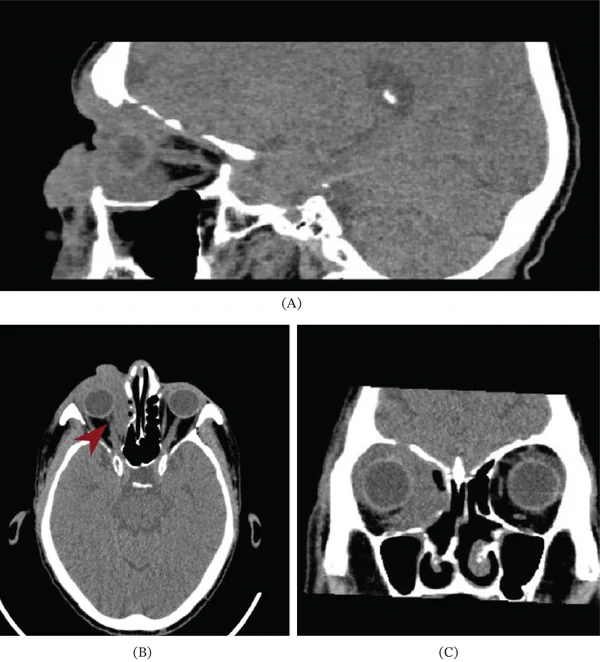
CT images of the patient, 7 months postsurgery. (A) Sagittal CT demonstrating extensive soft‐tissue tumor progression into the upper eyelid and superior orbital quadrants. (B) Axial CT showing a large anterior orbital mass with cutaneous/eyelid involvement. Red arrowhead highlights posterior intraorbital extension into the retrobulbar space. (C) Coronal CT displaying diffuse orbital infiltration across the superior, medial, and inferior quadrants with bone erosion and medial wall displacement into the ethmoid sinus.
